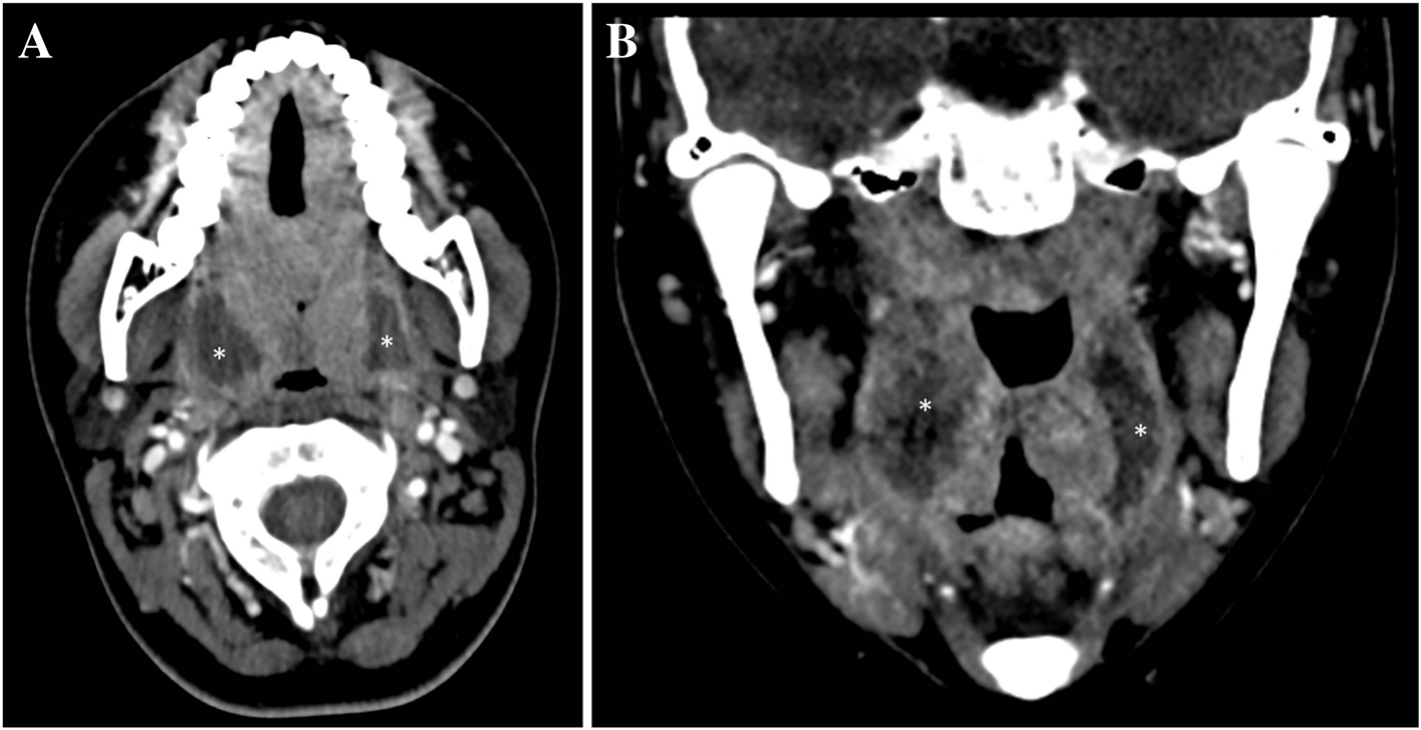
Horizontal (panel a) and coronal (panel b) CT-scan disclosing voluminous bilateral tonsillar abscesses (*asterisks*) in one of the patient included in the study who had consumed AID before hospital admission
